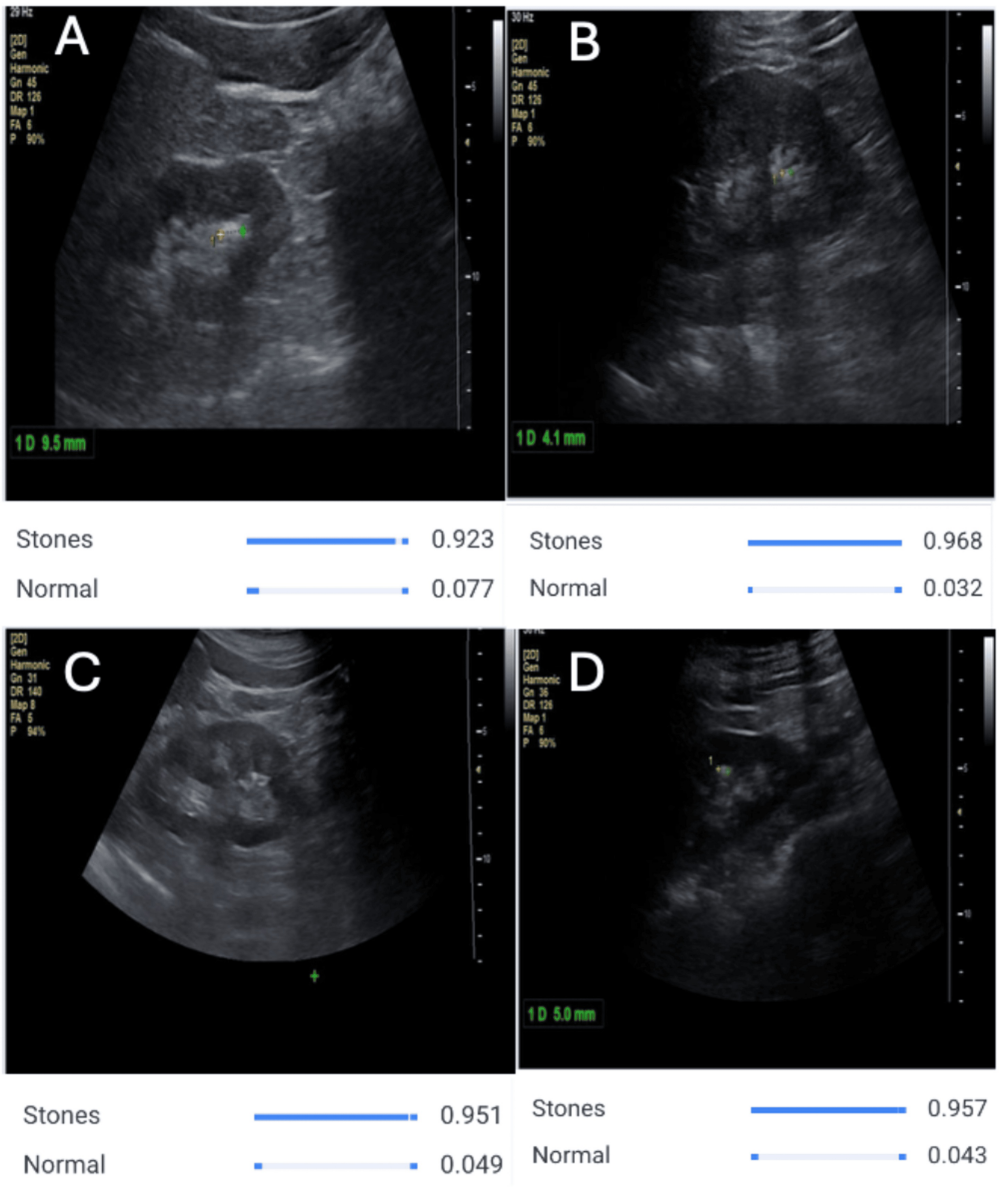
AI model detecting kidney stones on ultrasound images. Representative ultrasound scans demonstrating correct stone detection by the AI model. Panels A–D show distinct renal views in which the system consistently assigns high predicted probabilities to the “Stones” class (approximately 0.92–0.97) and low probabilities to the “Normal” class. These outputs align with visual sonographic features such as echogenic foci and posterior acoustic shadowing. Probability bars beneath each panel correspond to the model’s classification confidence for each labeled image (A–D).
